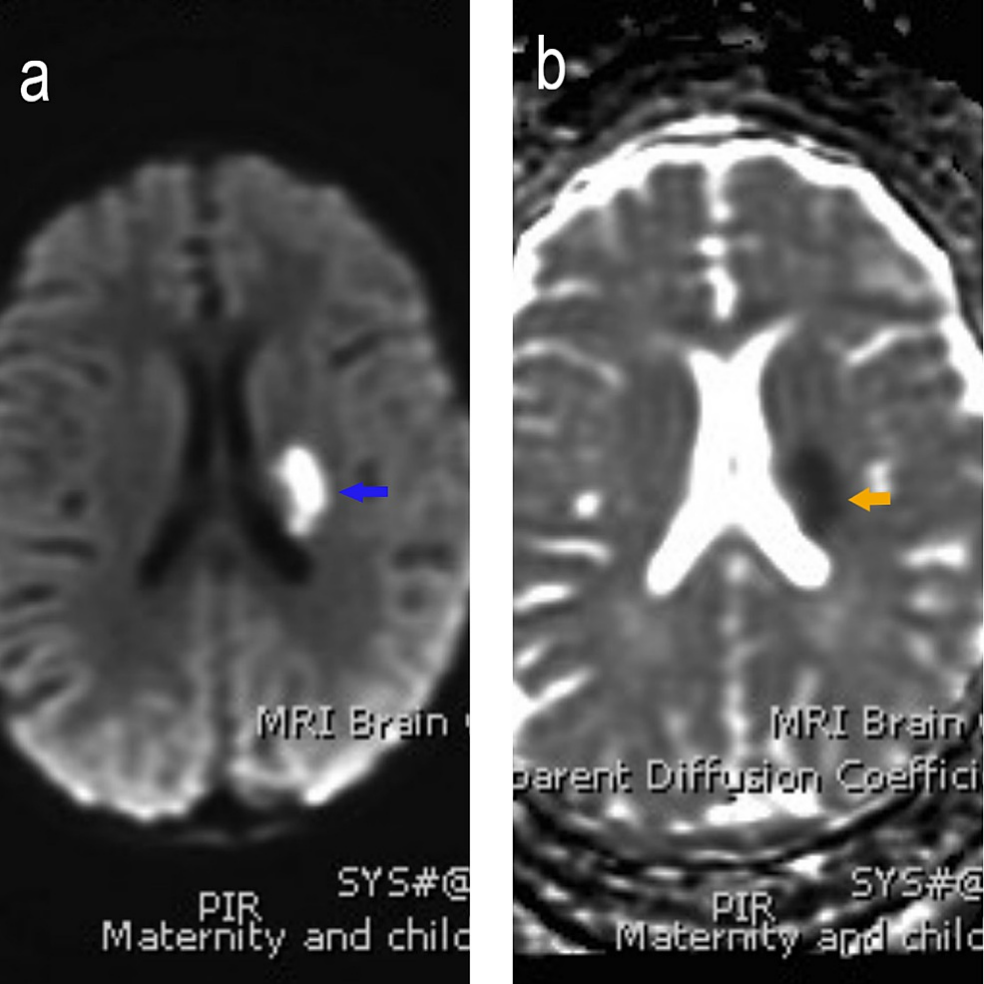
The MRI brain shows (a) bright signal (blue arrow) of the left basal ganglia on diffusion-weighted imaging (DWI), and (b) black signal (orange arrow) on the apparent diffusion coefficient map (ADC map), showing restricted diffusion, which indicates acute infarction.
